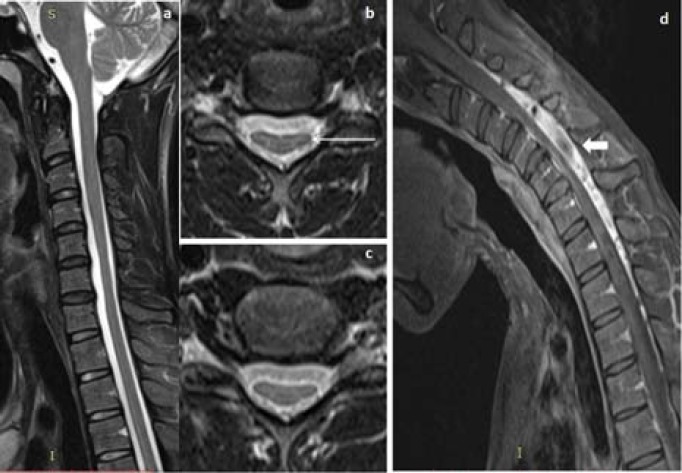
A 21-years old man with bilateral hand muscles weakness, neutral MRI sagittal T2WI (a) showed focal lower cervical cord atrophy without cord hyperintensities. Axial T2WI (b, c) showed anterior flattening of lower cervical cord (arrow), post-gadolinium sagittal flexion MRI T1WI (d) showed anterior displacement of posterior dura matter with enhancing posterior epidural lesion and T1 hypointense cerebrospinal fluid flow voids within (block arrow)
